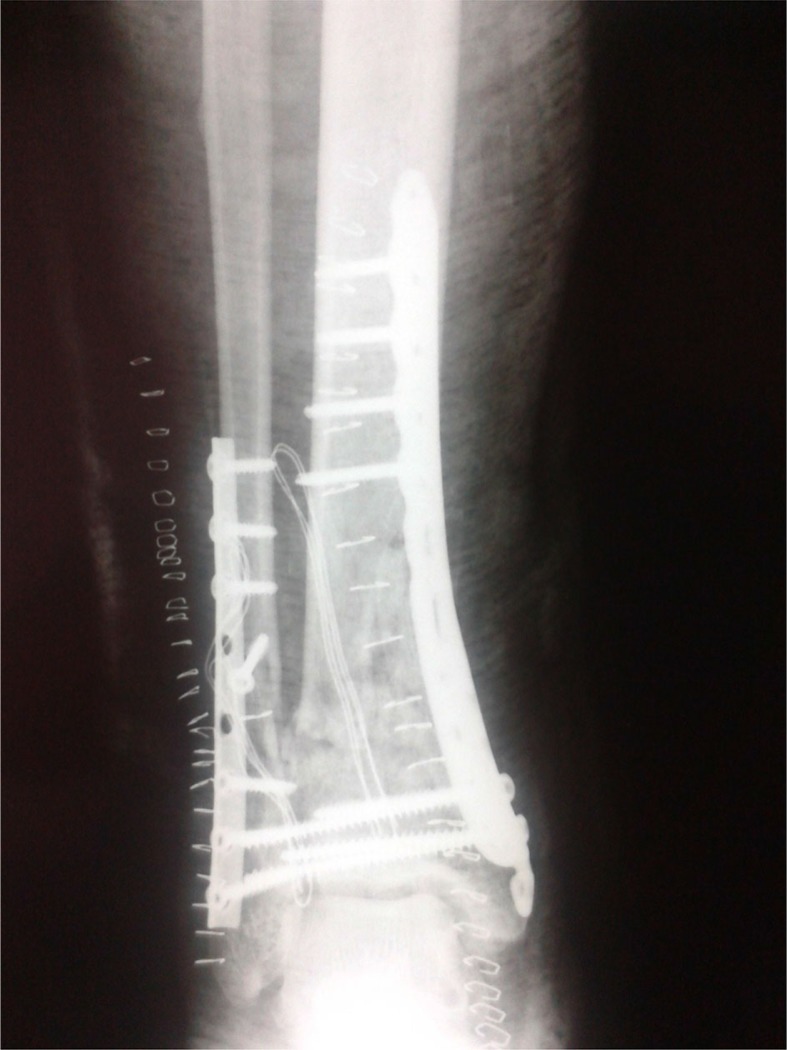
Postoperative anteroposterior lower extremity radiograph showing the internal fixation of the pilon fracture.
